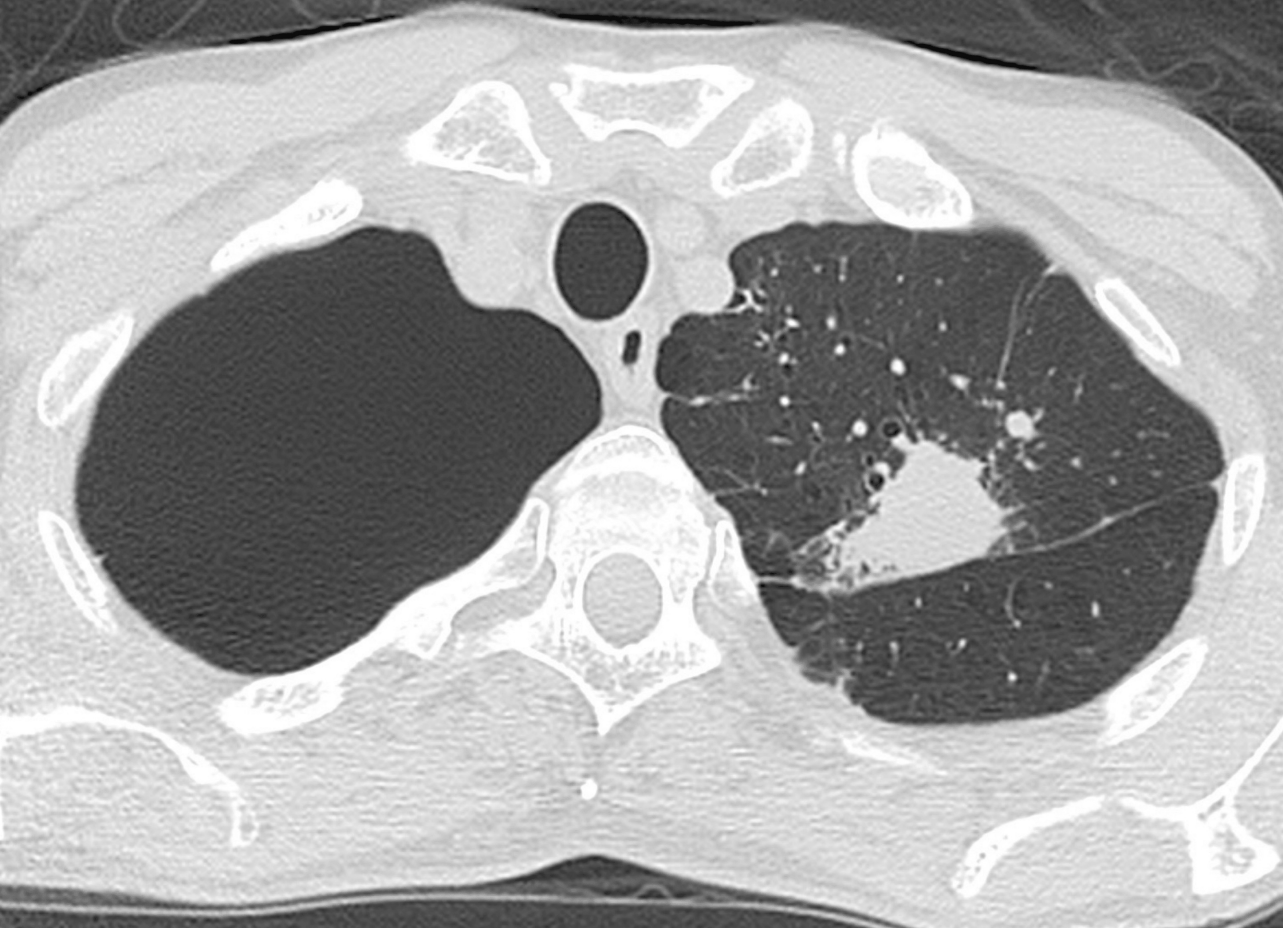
Preoperative CT scan Tumor located in the left upper lobe of the lung.
